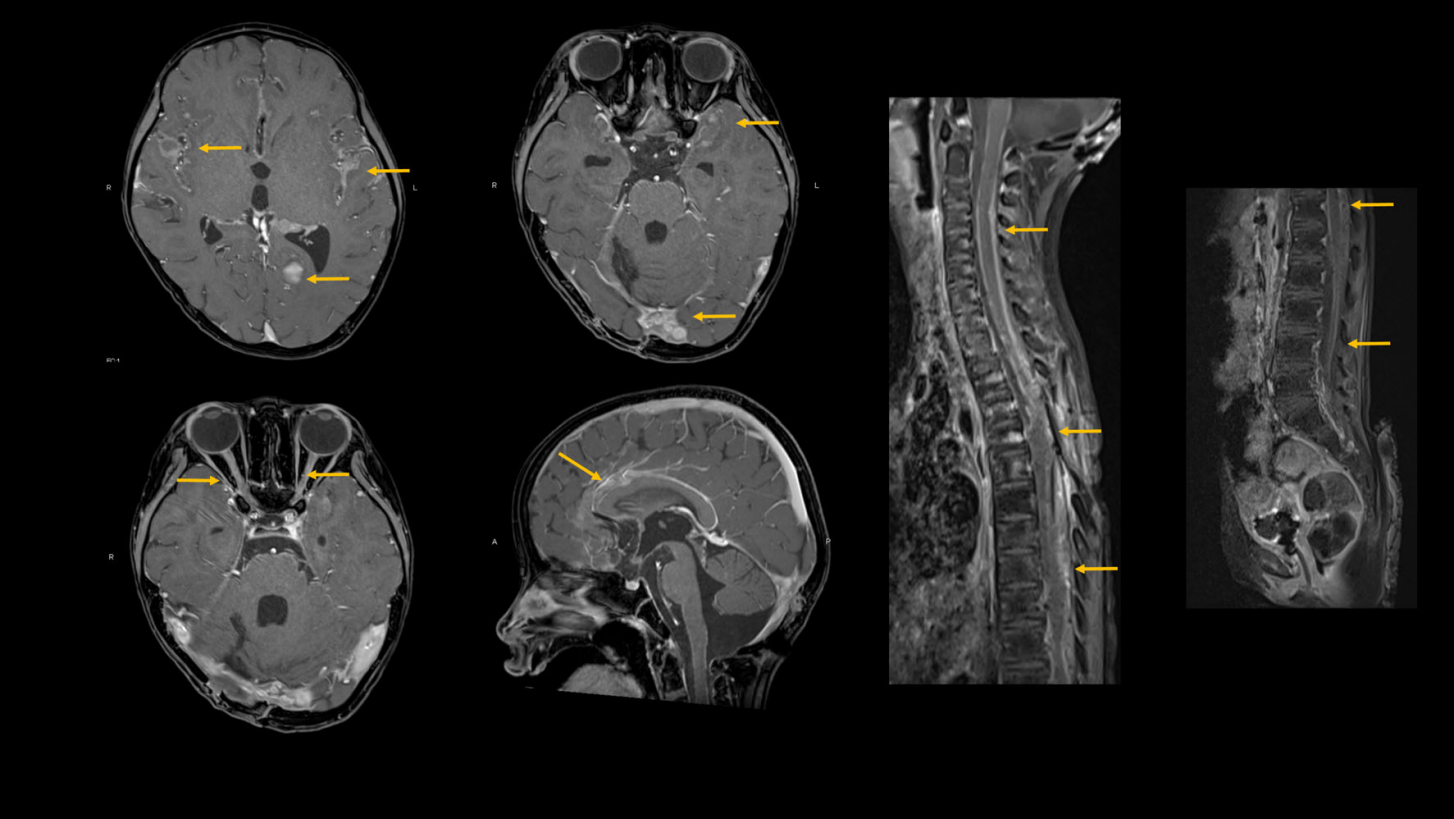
Gd‐T1w MRI revealed whole cerebrospinal dissemination and multiple metastases. Head MRI showed extensive contrast enhancement of the leptomeningeal membrane, with enhancement effects along the bilateral optic nerves. Spinal MRI revealed a diffuse enhancement effect within the spinal canal. There was an enhancing mass in the postoperative area of the upper thoracic spine and a continuous enhancing effect along the surface of the spinal cord to the spinal conchal level.
